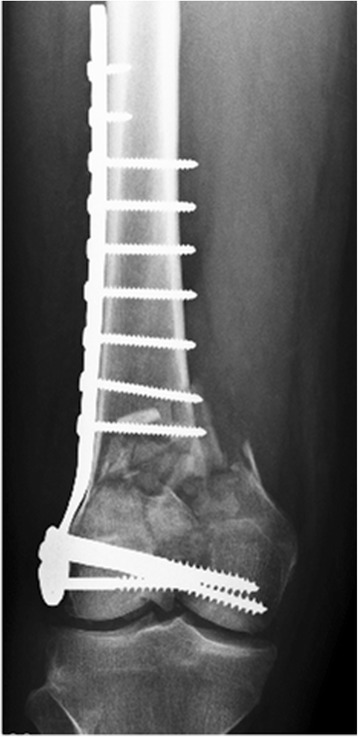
Anteroposterior radiograph of the right femur 3 days post-operatively
